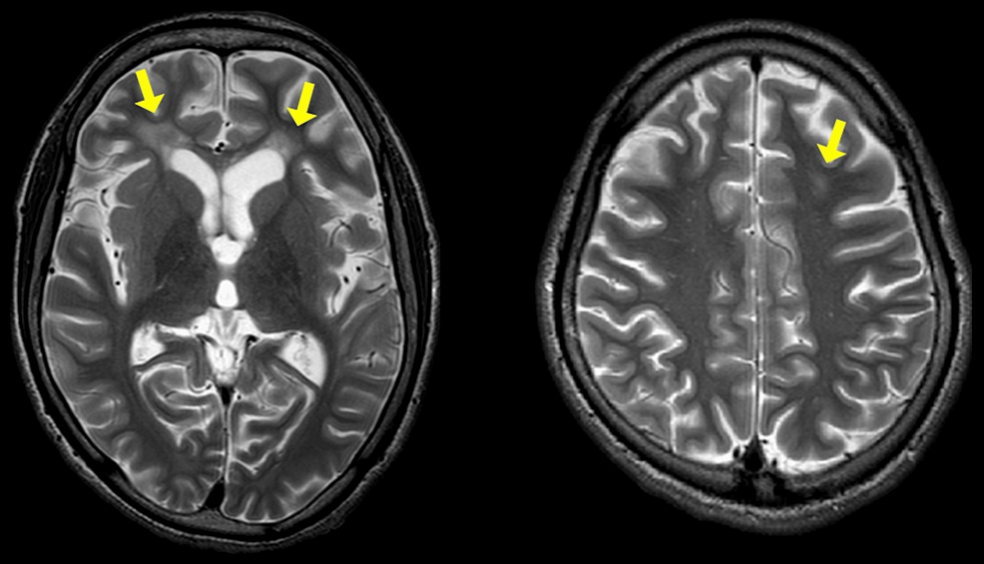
Magnetic resonance imaging findings before the initiation of immunotherapy. On T2-weighted images, diffuse cerebral atrophy and hyperintense lesions (arrows) in the periventricular white matter are observed.
